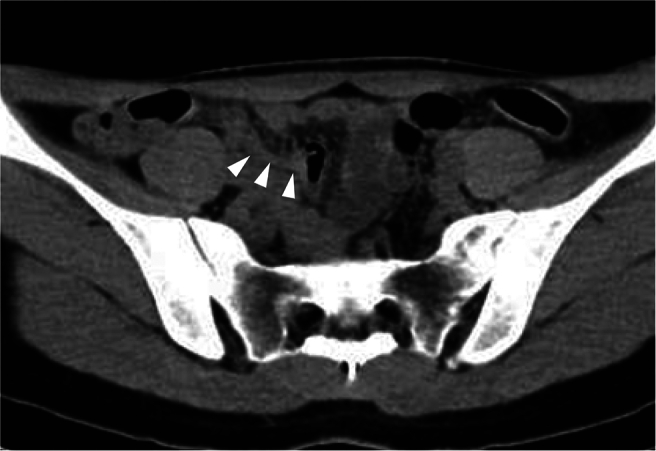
Enlarged appendix on CT scan. Reevaluation of the preoperative CT scan after laparoscopic surgery identifies an enlarged appendix (arrowheads).
